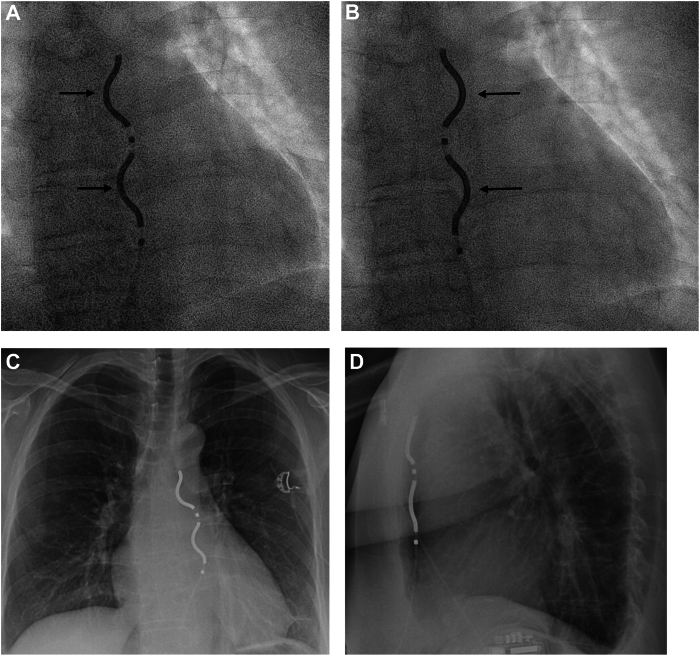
An example of flipping extravascular implantable cardioverter-defibrillator lead. A: Initial lead position immediately after introducer removal, with coils oriented to the right (*arrows*). B: The lead flipped 180 degrees within minutes, now with coils facing the left (*arrows*). The lead was removed and retunneled, aiming slightly more medially. C, D: Chest radiograph from the following day shows the final lead position near the sternum, with coils correctly oriented to the right. Note: These images are not associated with the reported cases.
